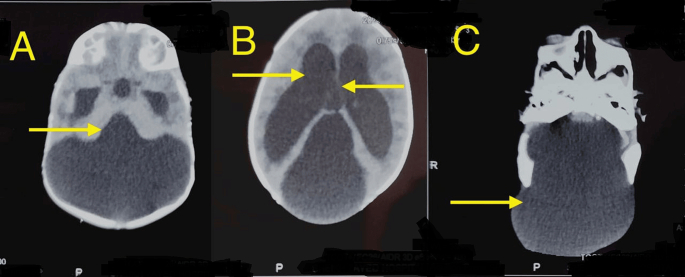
CT brain plain axial sections (A) shows large cystic area occupies the posterior fossa communicating directly with the fourth ventricle associated with vermian hypoplasia (representing Dandy walker malformation) and moderate dilatation of the lateral and third ventricles (B). This large cyst shows communication with an occipital encephalocele protruding through bone defect (C).
